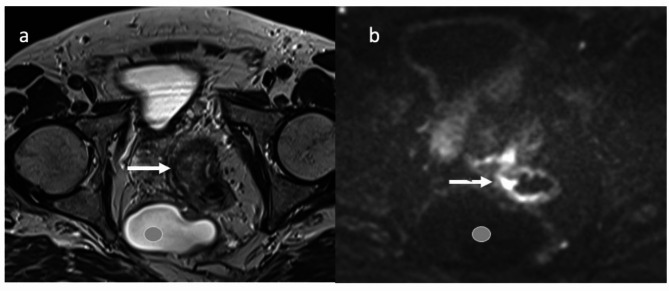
a Axial T2 images of the presacral cyst (grey circle) and the tumor at the dorsal circumference of the anastomosis. b Diffusion weighted MRI images at the same height of a with intense signal in the tumor at the anastomotic region
